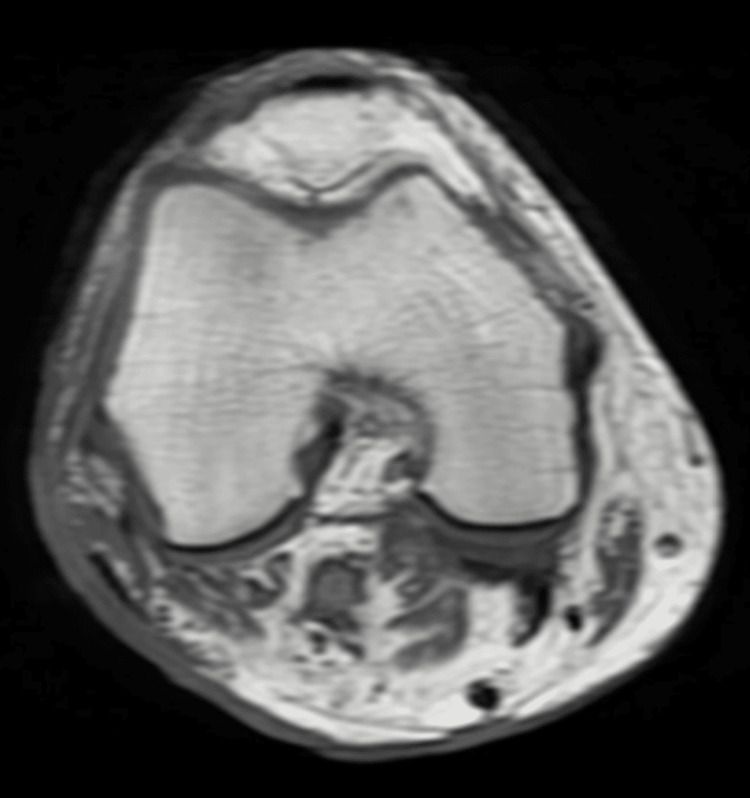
MRI findings of primary cutaneous anaplastic large cell lymphoma MRI of the right lower extremity demonstrating an ulcerated soft tissue lesion involving the lateral aspect of the distal thigh, with adjacent inflammatory changes and reactive-appearing regional lymphadenopathy. No evidence of deep muscular invasion or distant extracutaneous involvement was identified. MRI: magnetic resonance imaging
